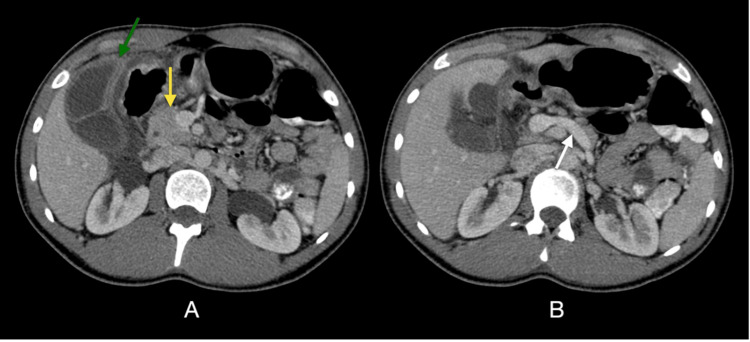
Acute cholecystitis in a case of complete agenesis of the dorsal pancreas Axial contrast-enhanced computed tomography (CECT) shows (A) a normal-appearing pancreatic head (yellow arrow), but neither body nor tail is present. This patient has a complete congenital absence of the dorsal pancreas and has additional gallbladder wall thickening (>3 mm) with mural/mucosal hyperenhancement for acute cholecystitis (green arrow). In (B), no pancreatic tissue is in the body-tail segments' expected position. There should normally be pancreatic tissue along the anterior aspect of the splenic vein (white arrow).
